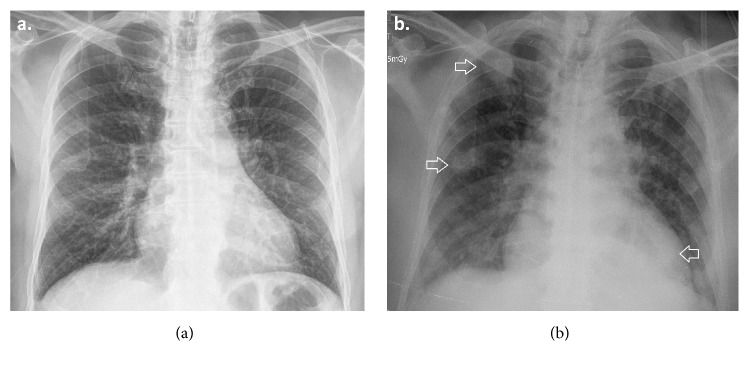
Chest X-ray. (a) Thickening of bronchial walls with no concomitant parenchymal abnormalities. (b) Multiple ill-defined lung nodules (arrows). Enlargement of the cardiac silhouette and left pleural fluid.
